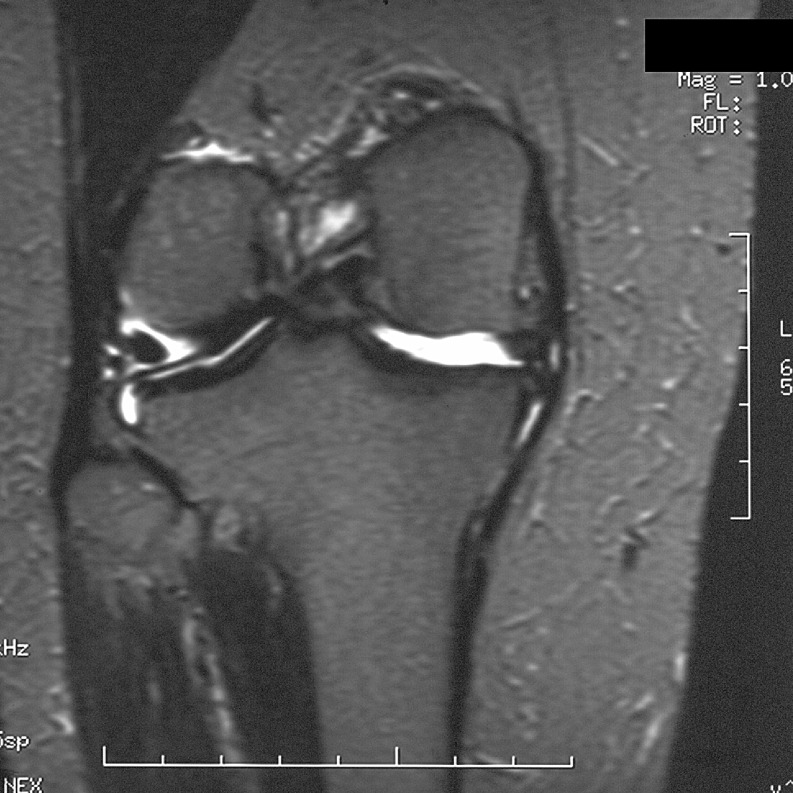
No bone marrow edema with a total joint replacement in a 66-year-old female patient. Coronal fast fat-suppressed T2-weighted image (TR 2500/TE 80/256 × 256) shows almost complete loss of hyaline cartilage in the medial compartment and an extruded, degenerative torn medial meniscus. Note the absence of marrow edema
